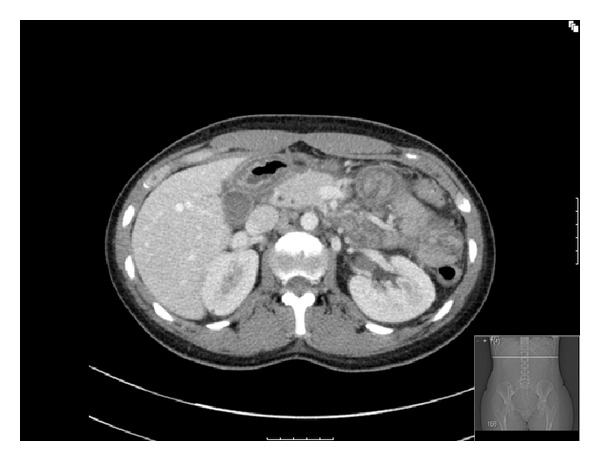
Abdominal computed tomography (CT) scan at admission indicated a thickened edematous bowel loop adjacent to pancreas with mild homogenous edema of pancreas.
